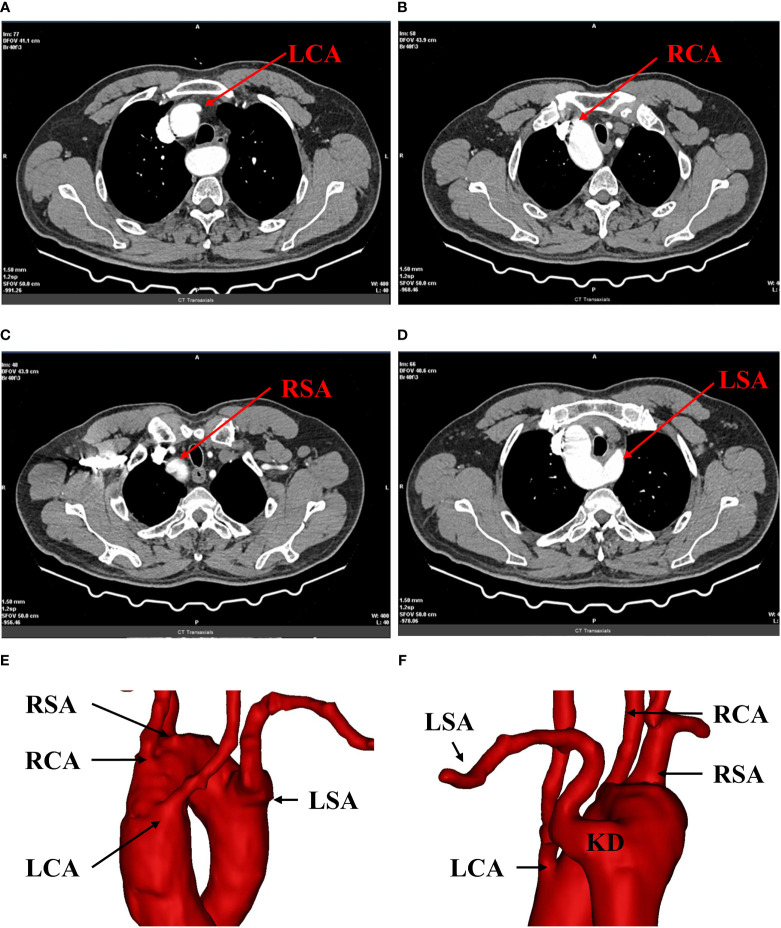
Preoperative 3D-CT reconstruction. (A) shows the opening of the left common carotid artery; (B) shows the opening of the right common carotid artery; (C) shows the opening of the right subclavian artery; (D) shows the opening of the left subclavian artery. (E, F) show the right aortic arch with branches and KD. LCA, left common carotid artery; RCA, right common carotid artery; RSA, right subclavian artery; LSA, left subclavian artery; RAA, right aortic arch; KD, kommerell diverticulum.
